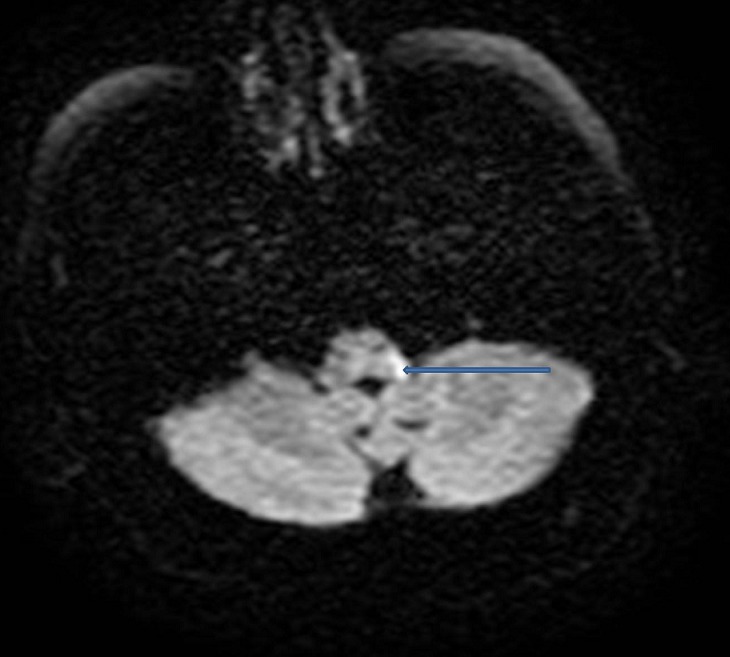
Diffusion-weighted axial MRI brain image showing the hyperintense
